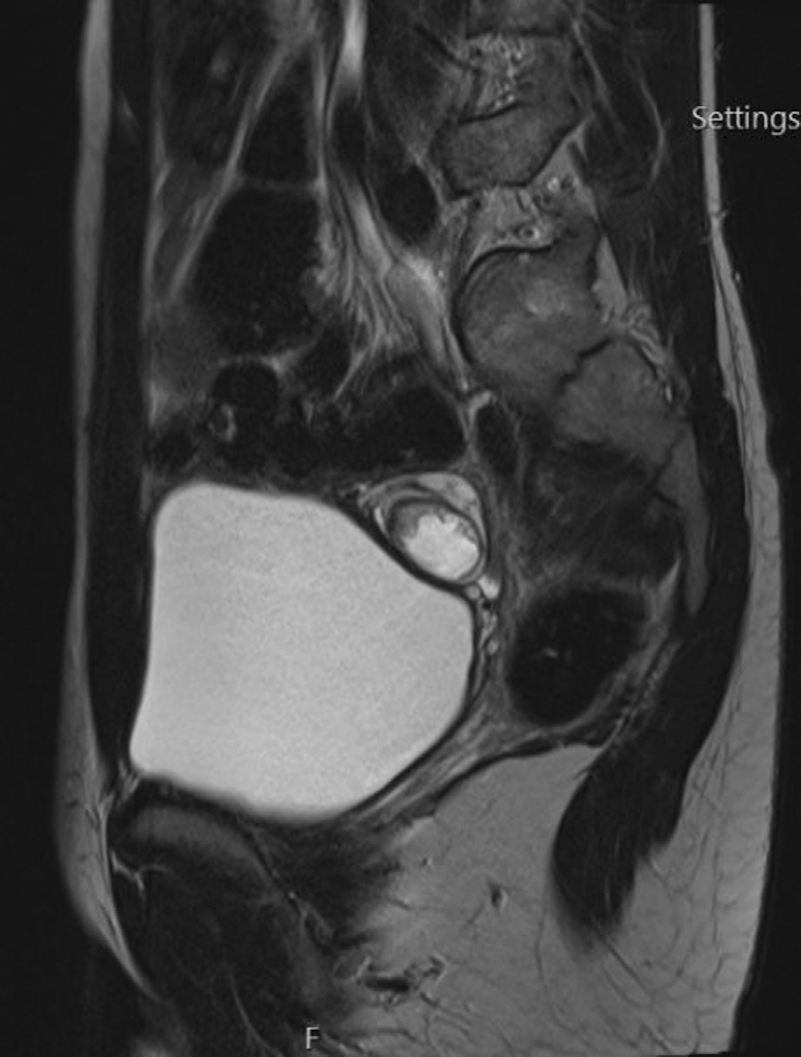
Magnetic resonance imaging (MRI) demonstrating a sagittal view of the metachronous left ovarian teratoma preoperatively.
